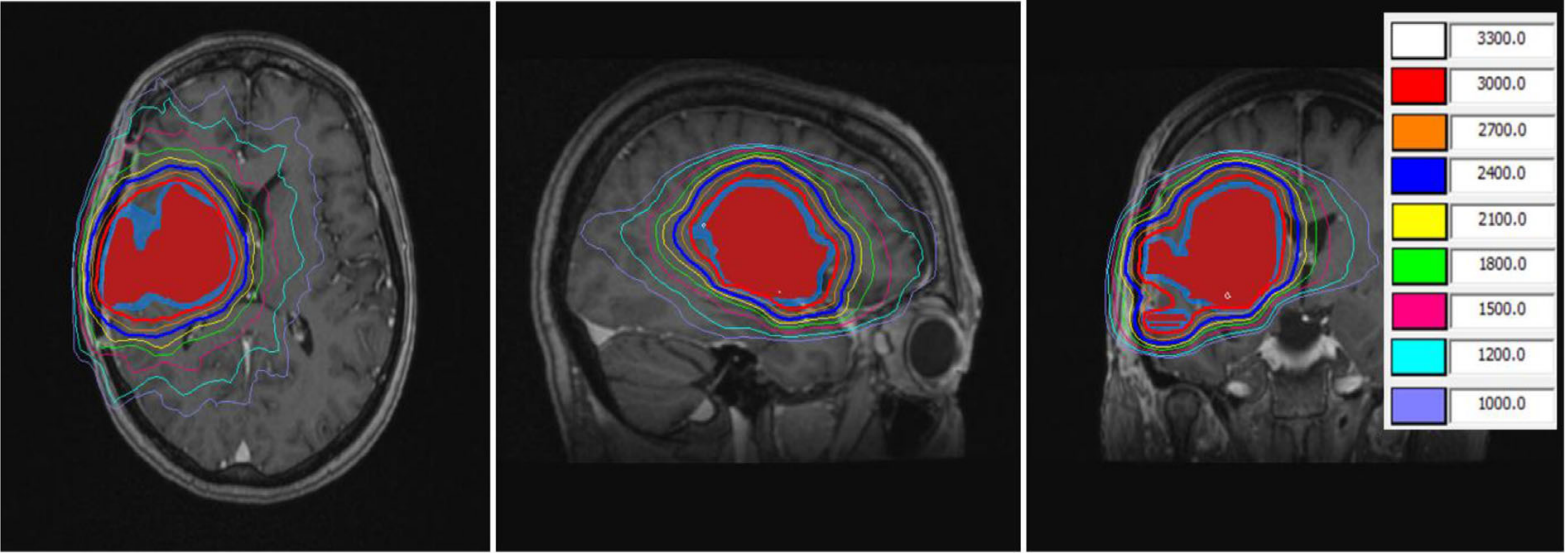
A patient magnetic resonance imaging (MRI) and Monaco reference plan. Red colorwash: GTV; blue colorwash: PTV. Isodose lines are in cGy
